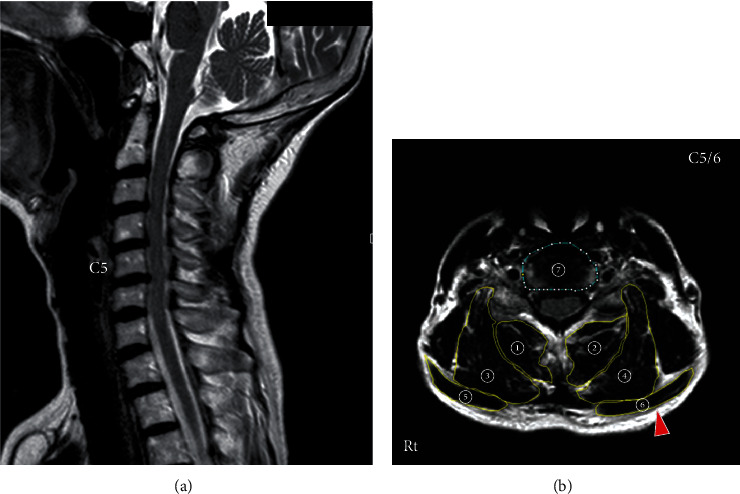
T2-weighted MR sagittal image (a) and axial image at C5-C6 (b) of the patient's cervical spine eight months after the first AR-PT. (a) Alignment of her cervical spine became normal. (b) Atrophy of her cervical extensor muscles was improved. Muscle tonus of the trapezius was restored (arrowhead). ➀➁: deep layers of cervical extensor muscles (semispinalis cervicis and multifidus). ➂➃: lateral-dorsal layers of cervical extensor muscles (splenius capitis, splenius cervicis, and longissimus). ➄➅: trapezius. ➆: C5-C6 disc.
